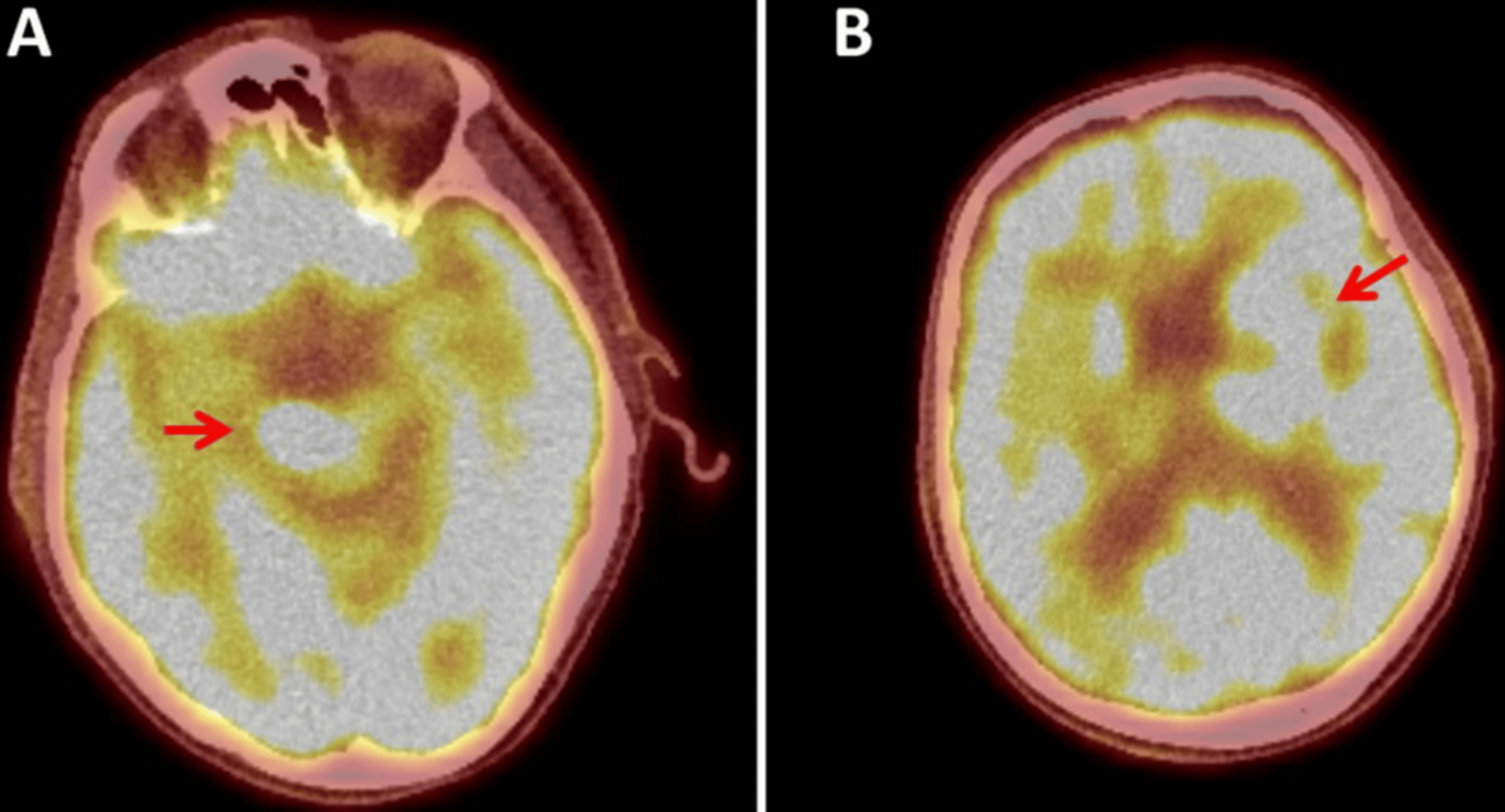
FDG-PET showing hypermetabolism in the right pedunculus cerebri (A) and the left basal ganglia (B). FDG-PET: fludeoxyglucose-18 positron emission tomography
